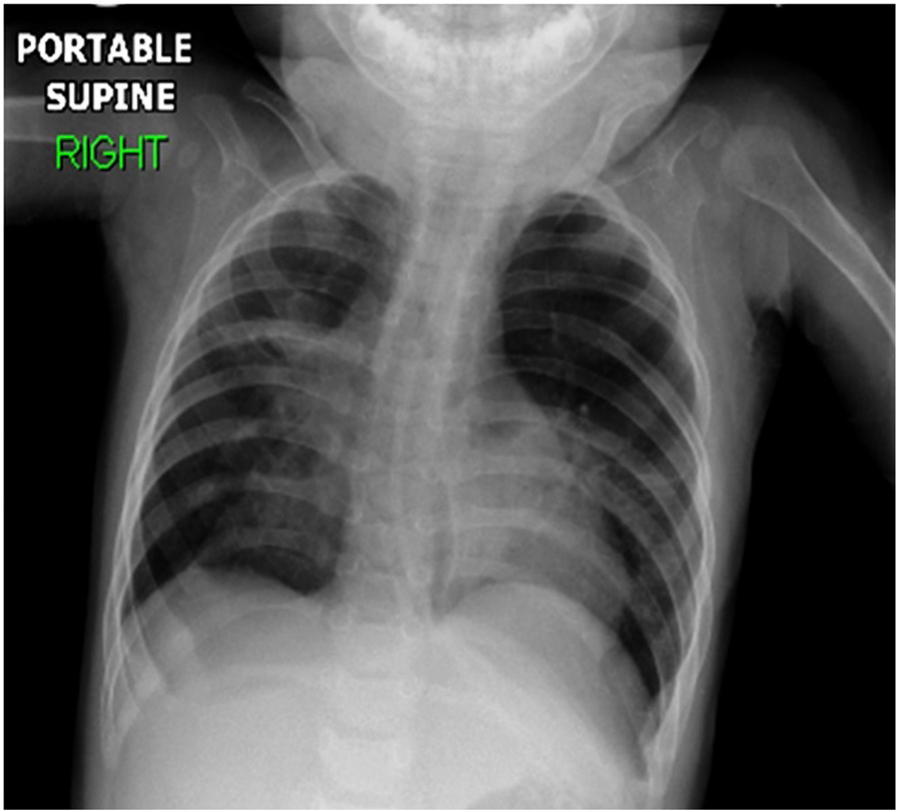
Chest X-ray anteroposterior view shows multiple subsegmental atelectasis, areas of air trapping and osteopenia
